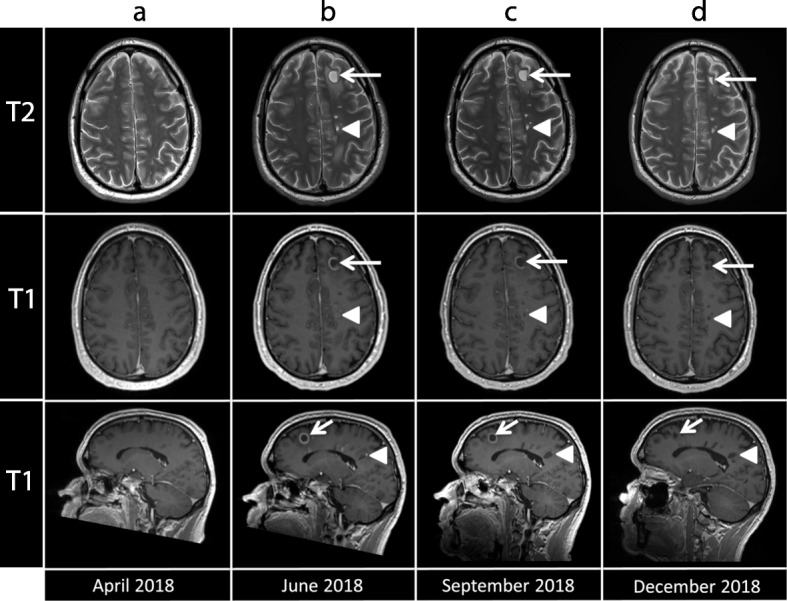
Display of demyelinating lesions evolution over 6 months. Successive brain MRI are displayed on axial T2-weighted images (top row), axial (middle row) and sagittal (bottom row) T1-weighted images after i.v. infusion of gadolinium contrast media. a Baseline in April 2018 before CNS demyelination. b Demyelinating lesions were diagnosed in June 2018. The largest lesion was located in the juxtacortical white matter of the left superior frontal gyrus (arrows) while numerous small ovoid lesions (arrows’ head) were located deep in the periventricular fronto-parietal white matter with a long axis perpendicular to the ventricle, corresponding to the typical “Dawson fingers” pattern. c After nivolumab discontinuation, lesions enhancement progressively decreased in September 2018. d The lesions disappeared in December 2018, leading to the characteristic “black hole” pattern on T1-weighted images
